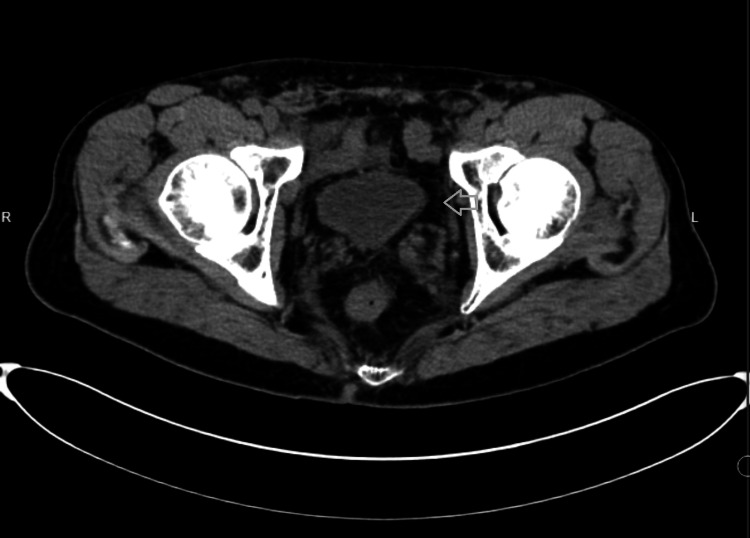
CT abdomen and pelvis without contrast (axial view) CT: computed tomography
